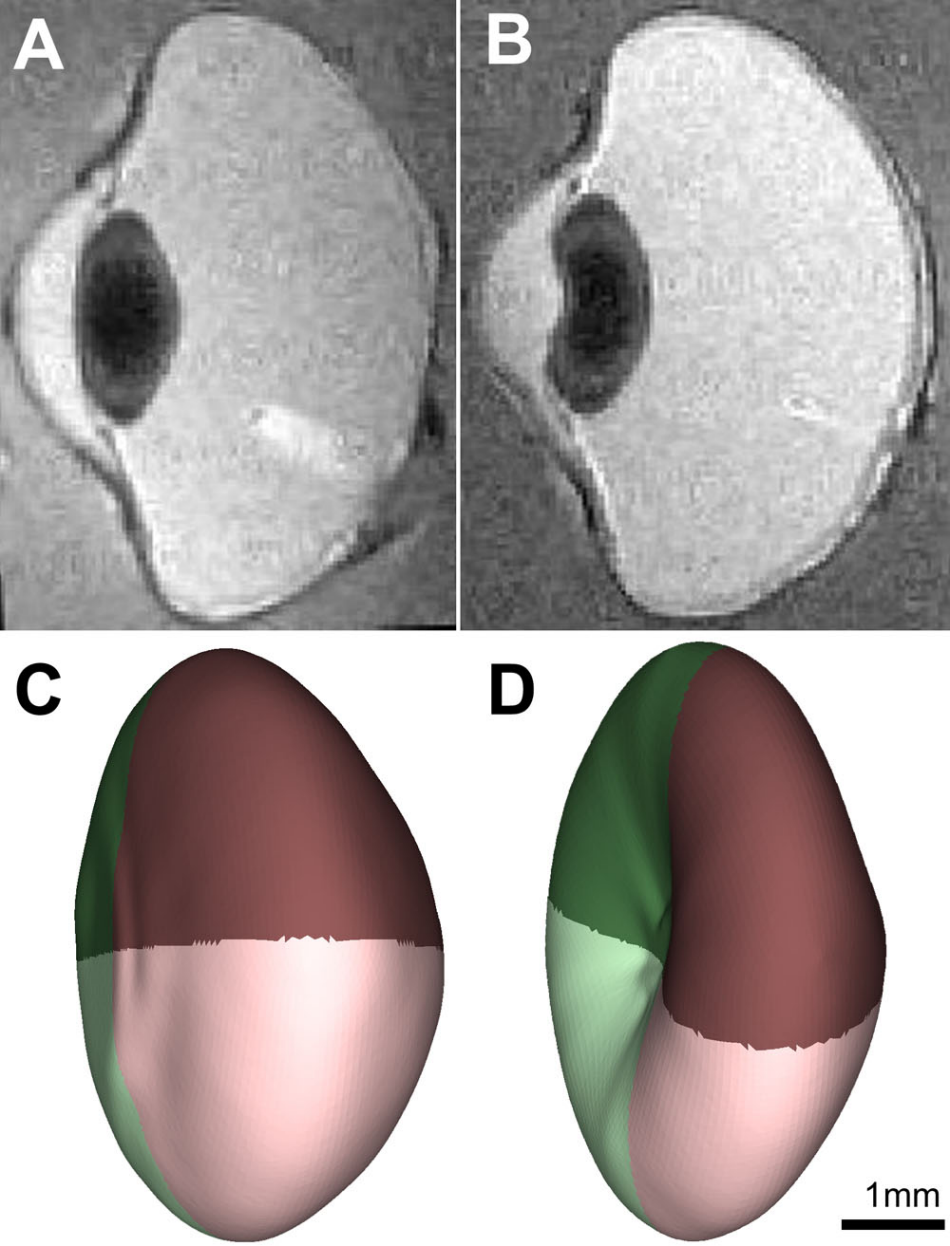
Typical appearance of kidney-shaped and biconvex  lenses. Panels A and B each show a single “slice” from the central region of a magnetic resonance imaging (MRI) scan: a nonkidney- shaped lens (A) and a kidney-shaped lens (B). Panels C and D show MRI3dX mesh models of the lenses from two eyes: a lens with a normal biconvex appearance (C) and a lens showing a depression the anterior surface, characteristic of kidney-shaped lenses (D). The scale bar in panel D is only an approximation since the true dimensions are altered due to the presentation in perspective.
